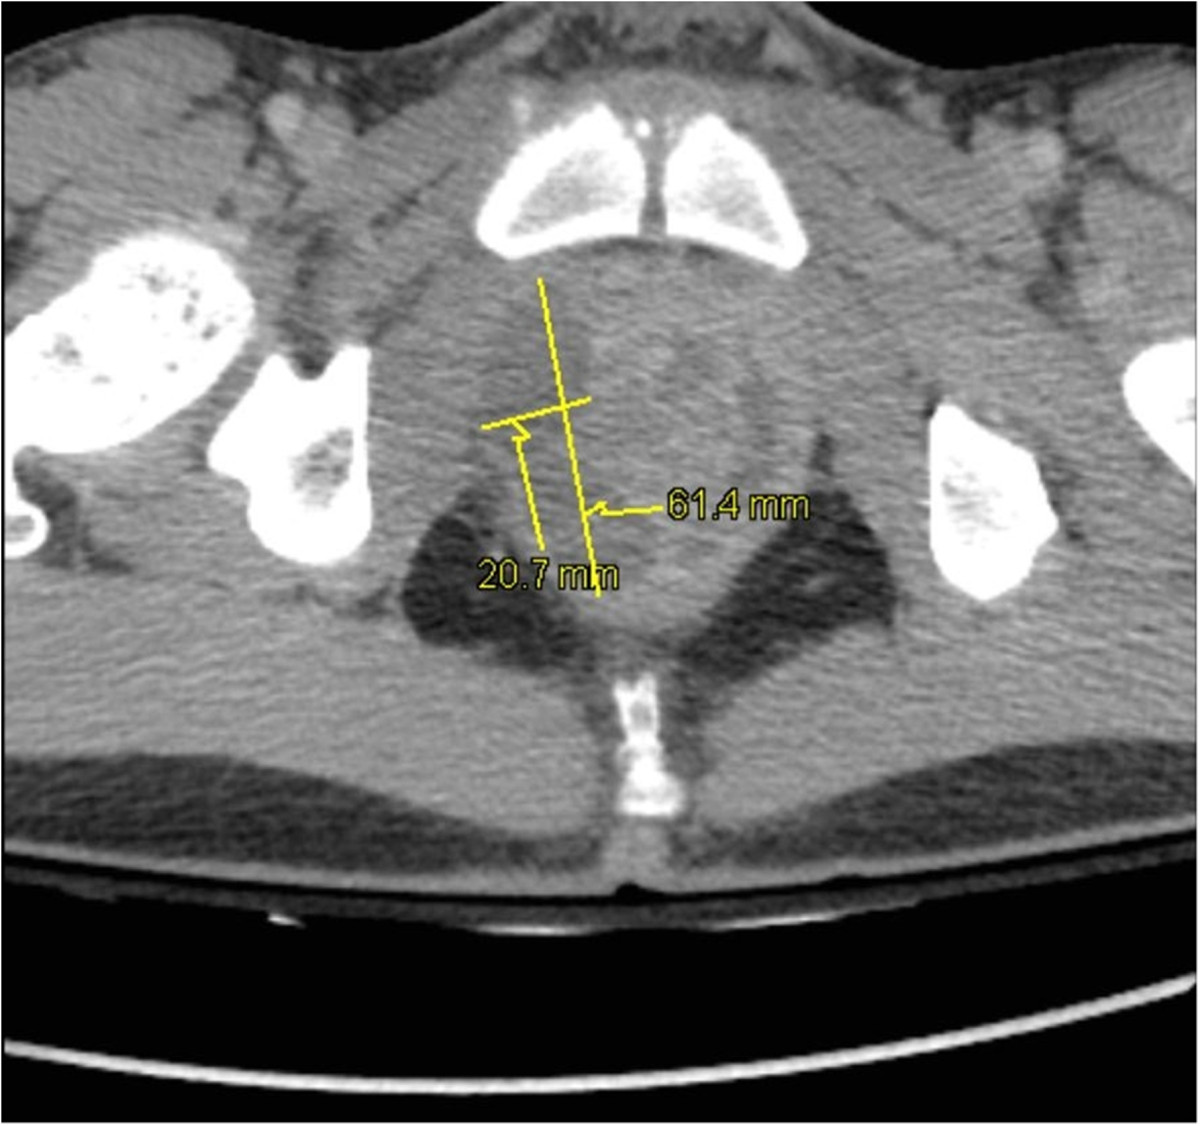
Pelvic CT-scan. Pelvic CT-scan showing prostatic hypertrophy and intra-prostatic abscesses: 20 × 15 × 33 mm and 64 × 21 × 26 mm in the right lobe and 38 × 10 × 30 mm in the left lobe.
